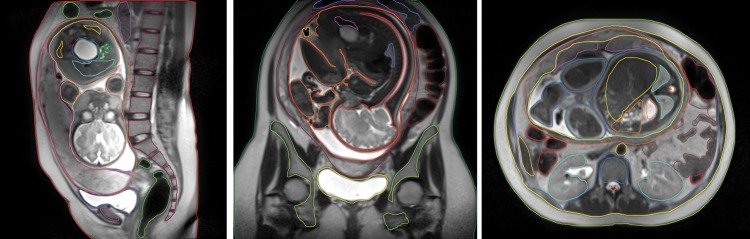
Segmentation of sagittal, coronal and axial MR image sets using 3D-Doctor software.
